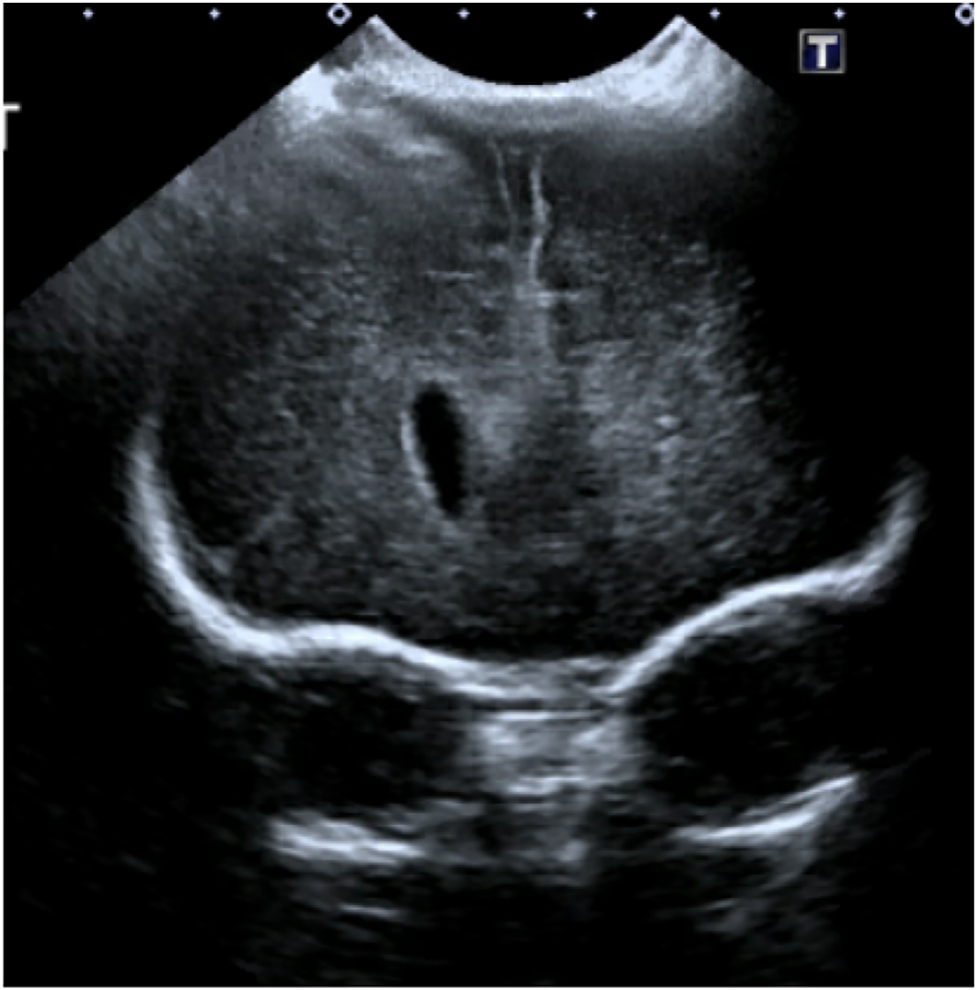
Head CT showed no abnormalities in extra-axial fluid space, periventricular white matter, and midline and posterior fossa structures. The ventricles were mildly enlarged but stable from prior examinations. Imaging also showed stable bilateral germinal matrix hemorrhages.
